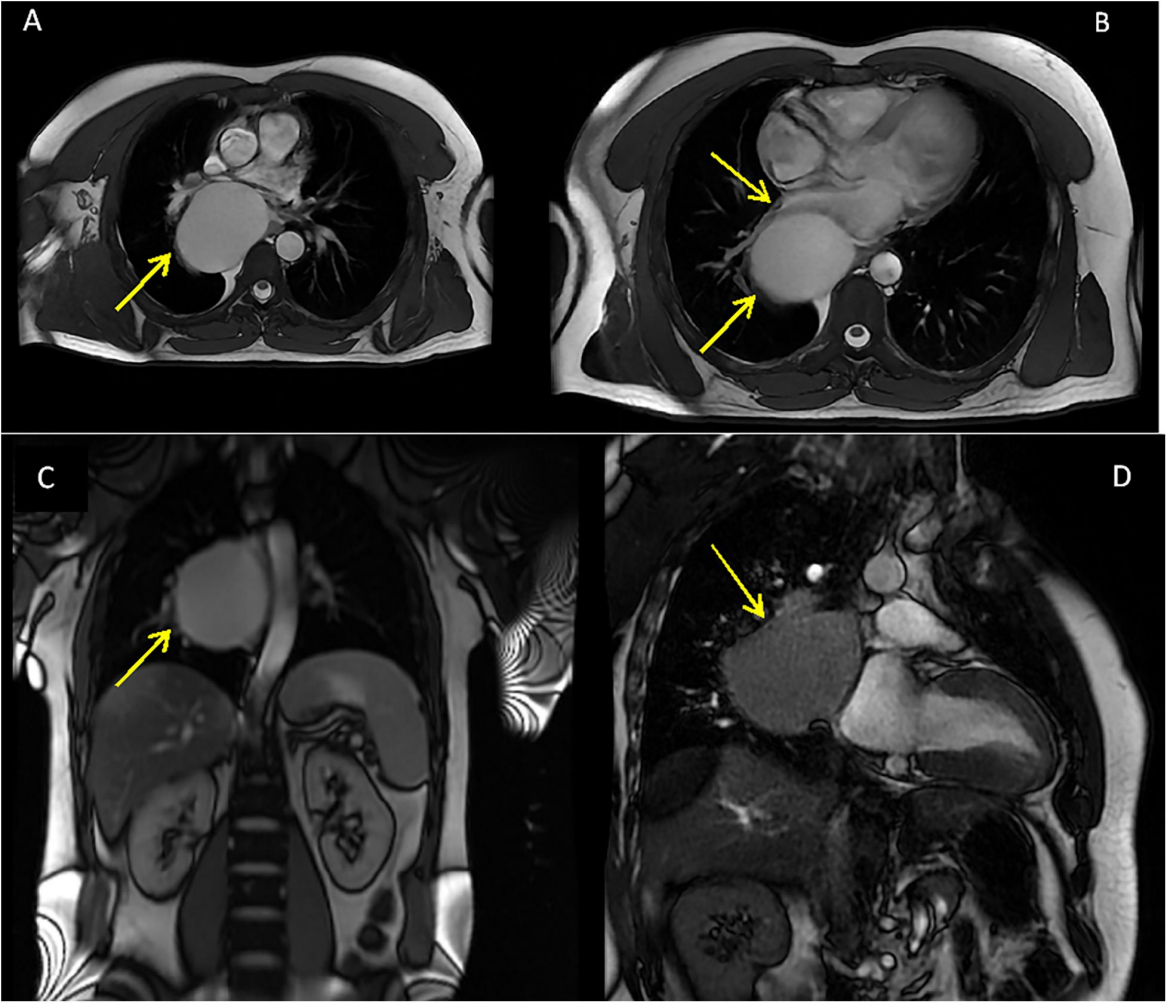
Cardiac magnetic resonance image with yellow arrows showing the oval formation: (A) Compression of the left inferior pulmonary vein. (B) Compression of left atrium and right inferior pulmonary vein. (C) Compression of the esophagus. (D) Position of the mediastinal cyst next to the left atrium.
